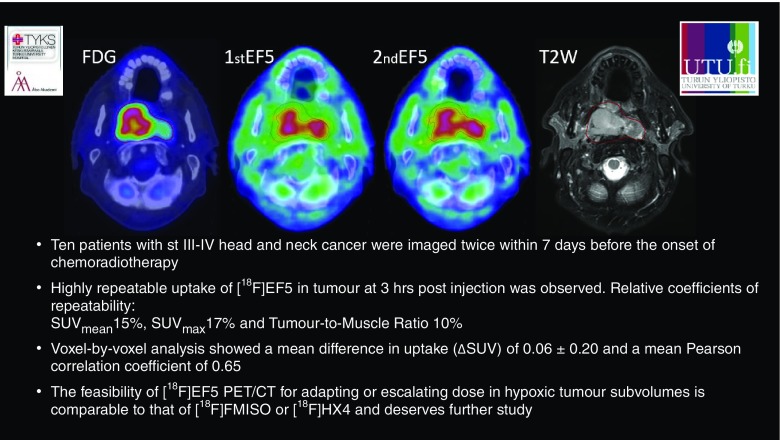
Repeatability of tumour hypoxia imaging using ^18^F-EF5 PET/CT in head and neck cancer [32]
